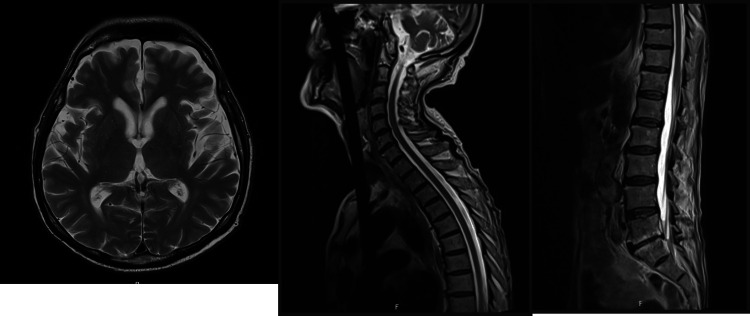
Normal MRI head and whole spine.
